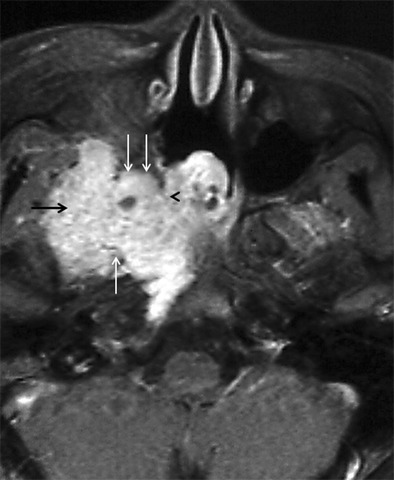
a JNA in a 17-year-old male who presented with recurrent epistaxis. Axial contrast-enhanced, FS T1W MR image shows a large, avidly enhancing mass in the right nasopharynx and PPF. The tumour extends from the right SPF (*arrowhead*) into the right PPF, expanding its walls (*thin white arrows*), and onwards laterally into the right ITF (*black arrow*)
